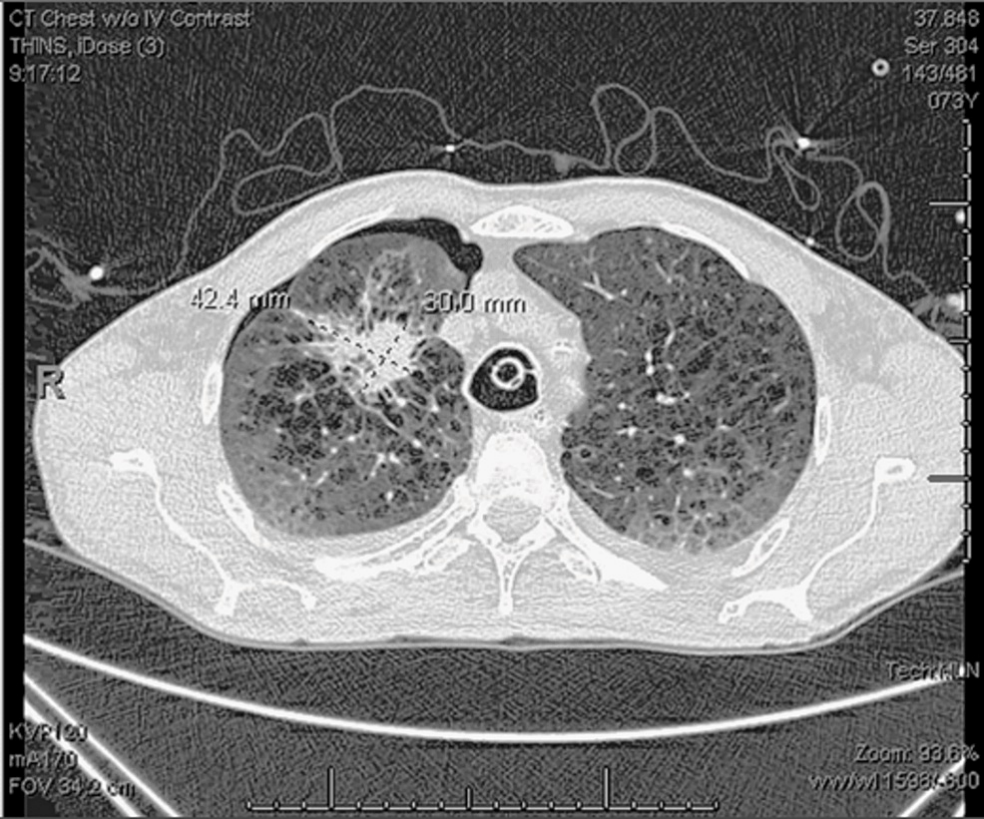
CT Scan of the Chest Following Eight Months of Treatment with Osimertinib This image was taken from a CT scan following eight months of treatment with osimertinib. The imaging study was conducted to monitor the progression of the disease during follow-up for the patient's lung tumor diagnosed eight months ago. The mass shown here corresponds to the original right upper lung mass detected on diagnostic imaging eight months ago. This imaging confirmed the growth of the original lesion and the progression of the disease, indicating that the malignancy was not responding to treatment and continuing to progress. Following this scan, osimertinib was increased from 80 mg to 160 mg to stop the progression of the disease.
